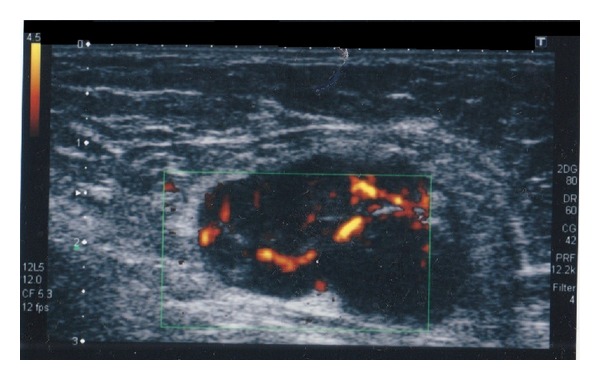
Ultrasonography of the left axillary shows an enlarged lymph node of about 35 mm with vascular poles at color-Doppler.
